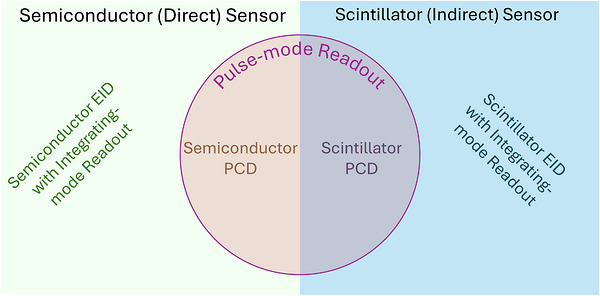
The fundamental distinction between photon‐counting detectors (PCDs) and energy‐integrating detectors (EIDs) lies in the signal readout paradigm. Most PCDs developed for CT applications employ semiconductor sensors; however, semiconductor sensing is not a fundamental requirement for photon counting, and scintillator‐based photon‐counting implementations are also under investigation for potential CT applications.
